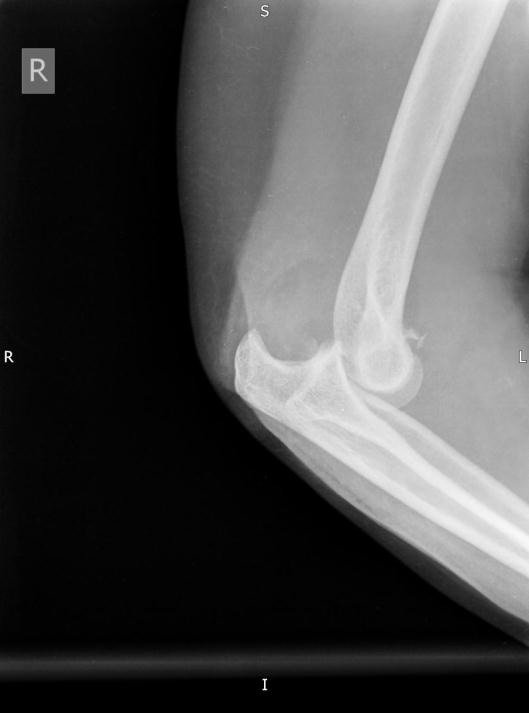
Terrible triad injuries may appear benign on plain radiographs.
